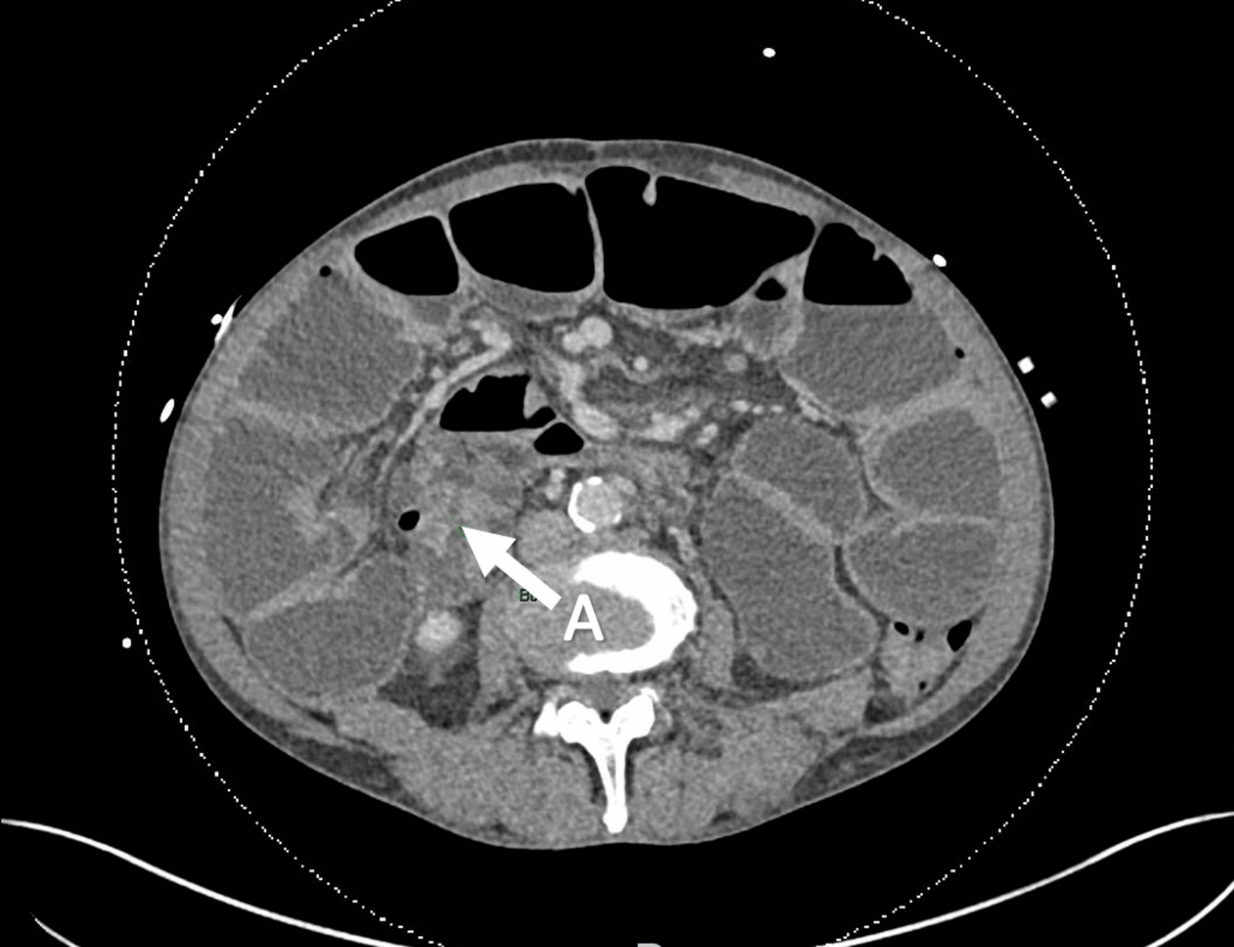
Admission 2 - axial view of CT scan showing apparent transition point at the distal terminal ileum. (A) Characterized by collapsed distal small bowel and tapering.
